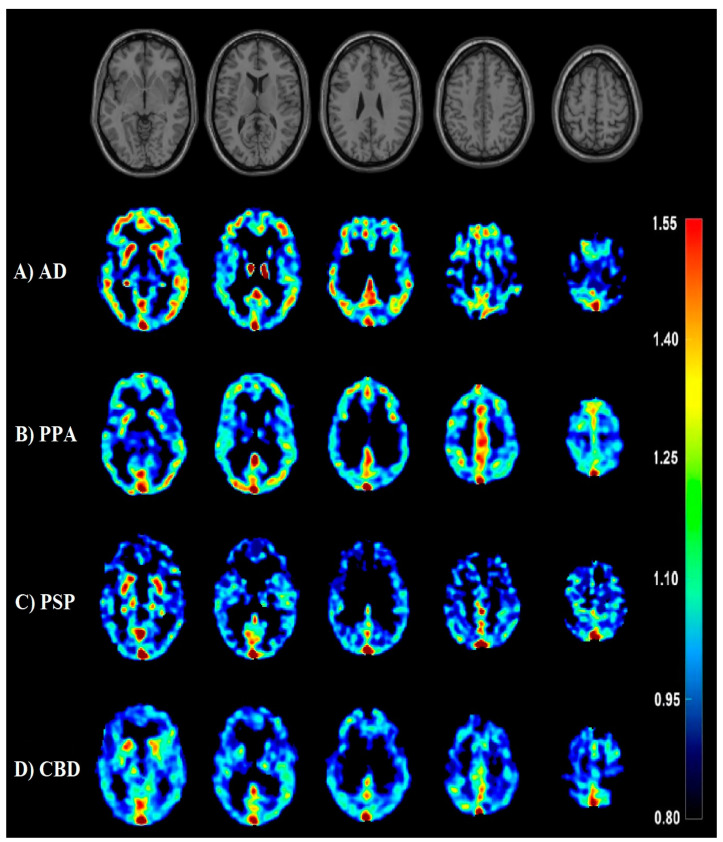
Serial transverse brain sections from [11C]PBB3-PET for a patient with (A) Alzheimer’s disease (AD: 73 years old, MMSE = N/A), (B) logopenic variant primary progressive aphasia (IvPPA: 69 years old, MMSE = 14), (C) a patient with progressive supranuclear palsy (PSP: 56 years old, MMSE = 27), (D) a patient with corticobasal degeneration (CBD: 52 years old, MMSE = 30). The single-subject T1-weighted structural template supplied with SPM12 is also presented to provide a detailed view of the brain structures. Note the off-binding targets in slices A–D, like the dural sinus, the plexus choroideus, and the basal ganglia. Department of Nuclear Medicine. 2023. “Axial slices in mixed dementia with C11-PBB3”. Department of Nuclear Medicine, University Hospital Ulm.
